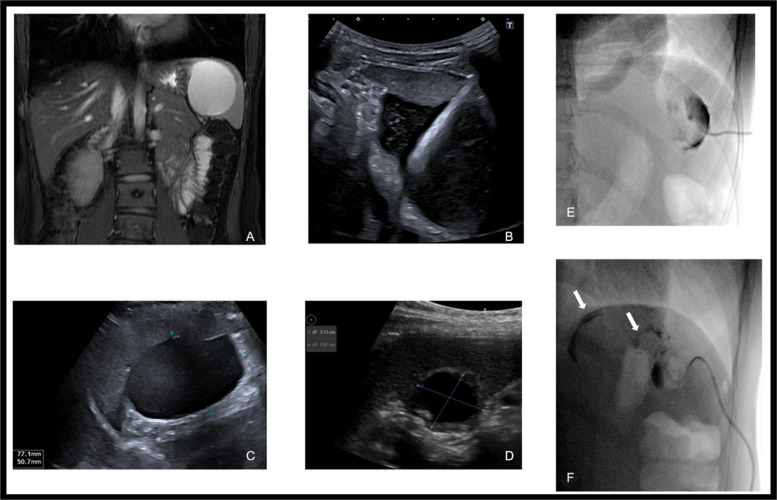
Different examples of sclerotherapy. A Large splenic cyst on coronal MRI. B Ultrasound guided insertion of Seldinger pigtail drain. C Cyst before treatment measuring 7.7 × 5.1 cm. D Cyst 4 months post one session of ethanol sclerotherapy measuring 2.2 × 2.5 cm. E Fluoroscopic contrast injection confirming drain within cyst before ethanol injection. F Different patient with splenic cyst – white arrows demonstrate contrast leaking out of cyst, thus unsafe for ethanol injection. Doxycycline was utilised instead in this patient
